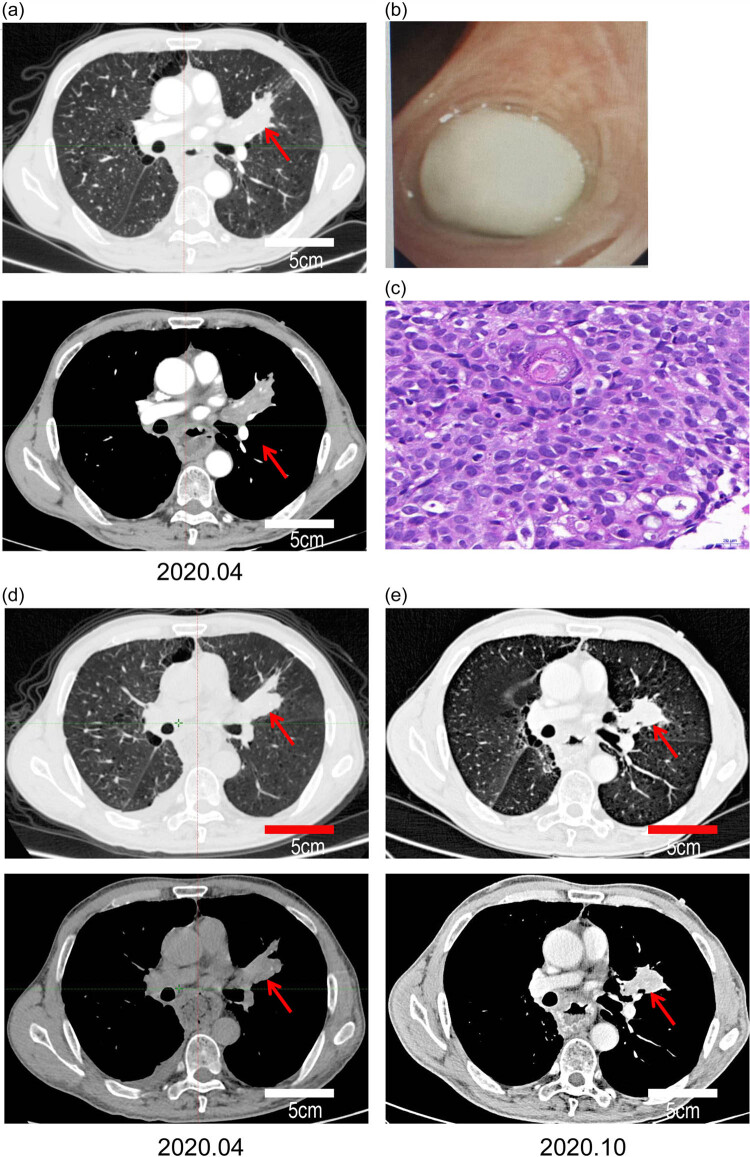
Observation of LUSC: (a) enhanced chest CT scan findings of left lung mass, contrast medium was iohexol, (b) findings of fiberoptic bronchoscopy (mass in the lingual segment of the left upper pulmonary lobe), (c) pathologic findings of the LUSC, and (d and e) chest CT scan of the LUSC after treatment with apatinib, (e) enhanced chest CT, contrast medium was iohexol.
